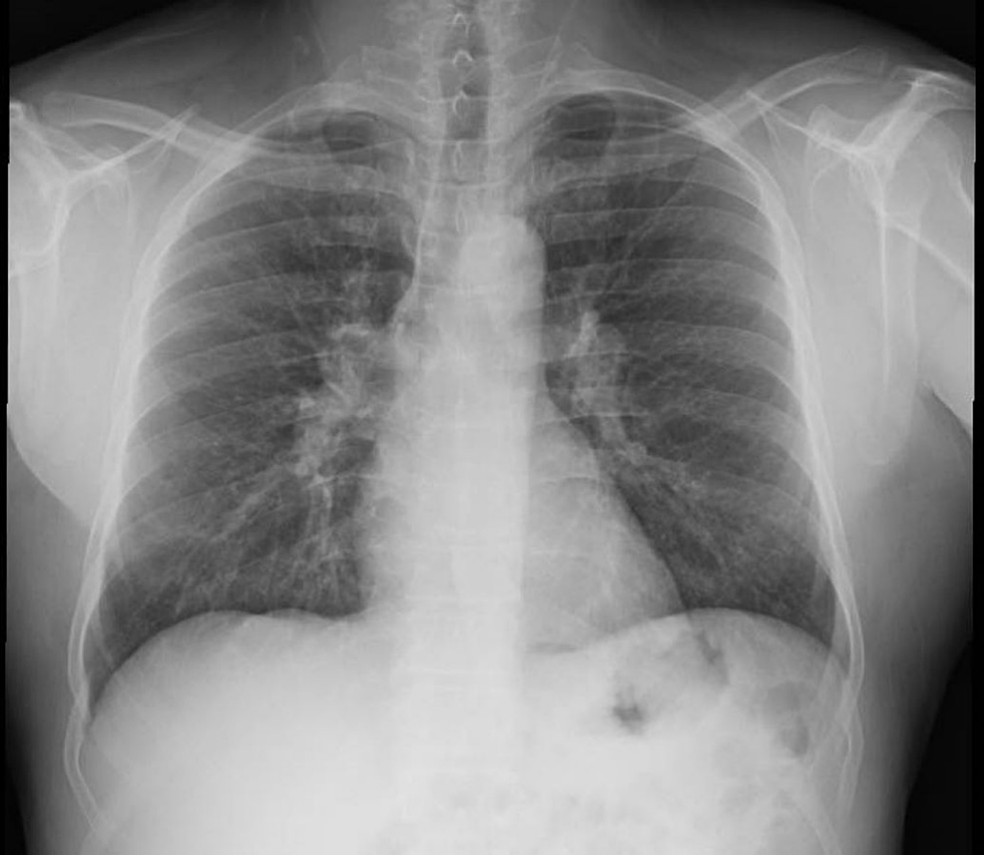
Chest x-ray (reevaluation) Reevaluation chest x-ray (three months later) showing clear radiological improvement.
